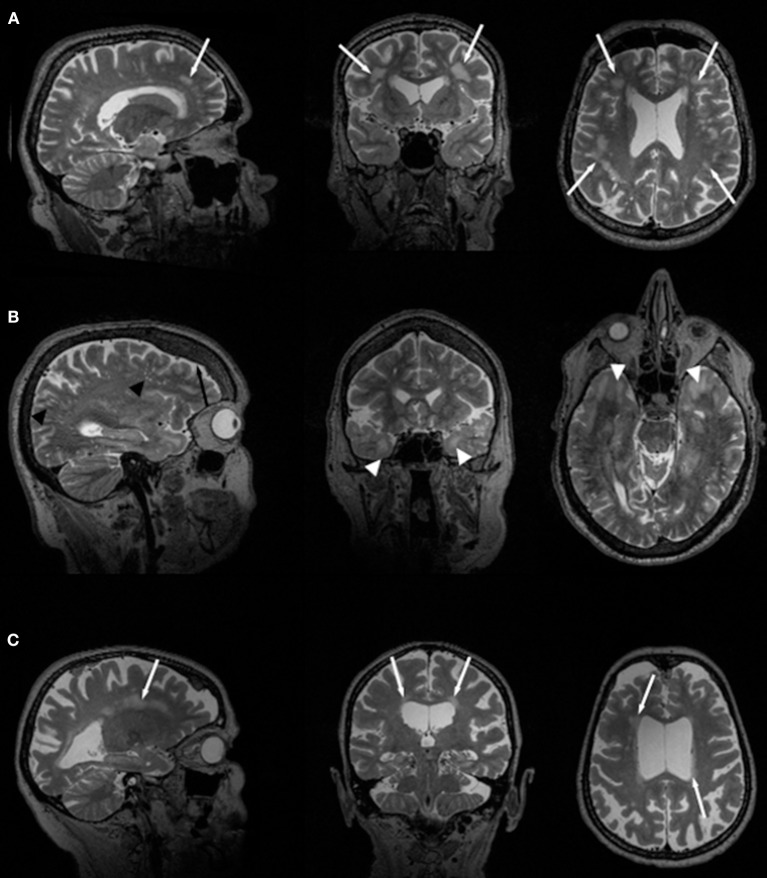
Slices of T2-weighted MR images in two different DM1 (A, B) and one DM2 (C) patients. Global atrophy with ventricle enlargement is seen in all cases. White arrows indicate WMHL, white arrow heads point toward ATWML. Black arrow indicates frontal hyperostosis in DM1 patients, black arrow heads point toward dilated Virchow-Robin spaces.
